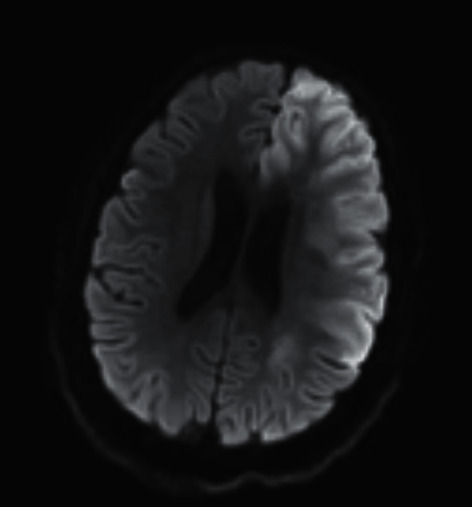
MRI brain without contrast performed during the patient's acute presentation to the emergency department showing large territory left hemispheric stroke.
